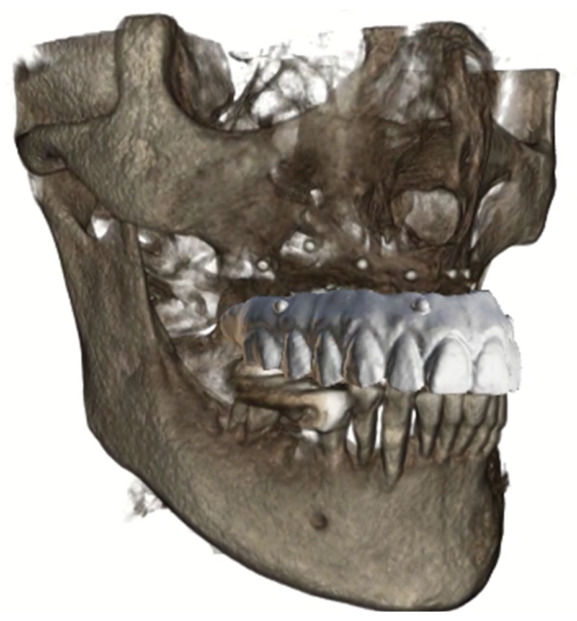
Registration of the CBCT, radiographic-template, prosthetic, soft-tissue, and occlusal datasets to create the integrated virtual patient and perform prosthetically driven implant planning.
